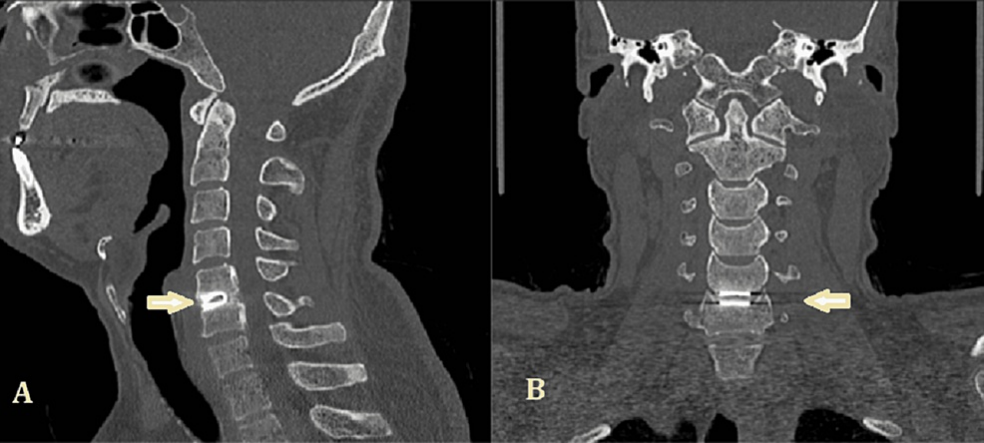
Sagittal (A) and axial (B) CT images of a patient with DCI (arrows) DCI, dynamic cervical implant
